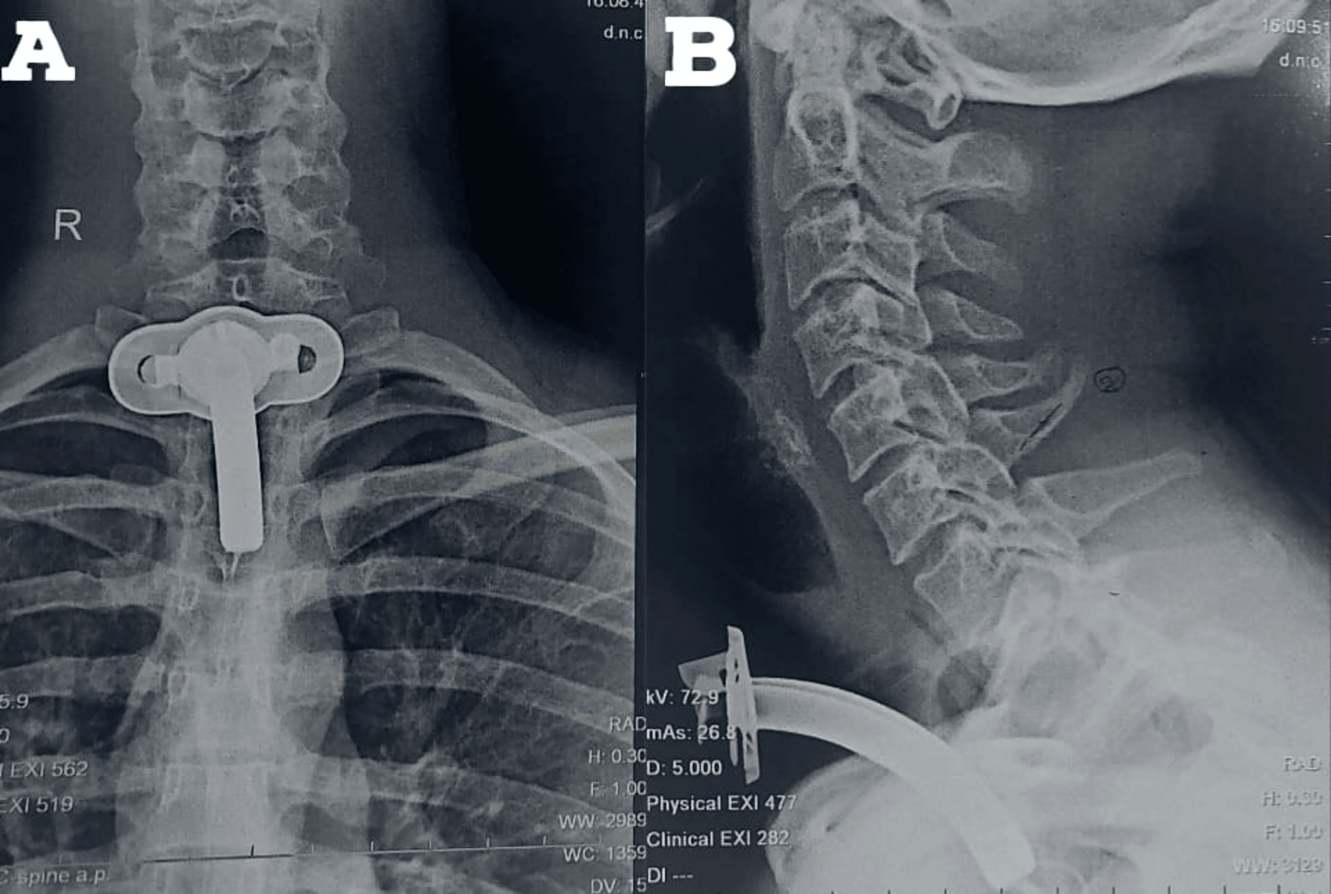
(A) X-ray cervical spine AP; (B) X-ray cervical spine lateral X-ray of the cervical spine showing tracheostomy site below C7 level, where the trachea is stenosed
